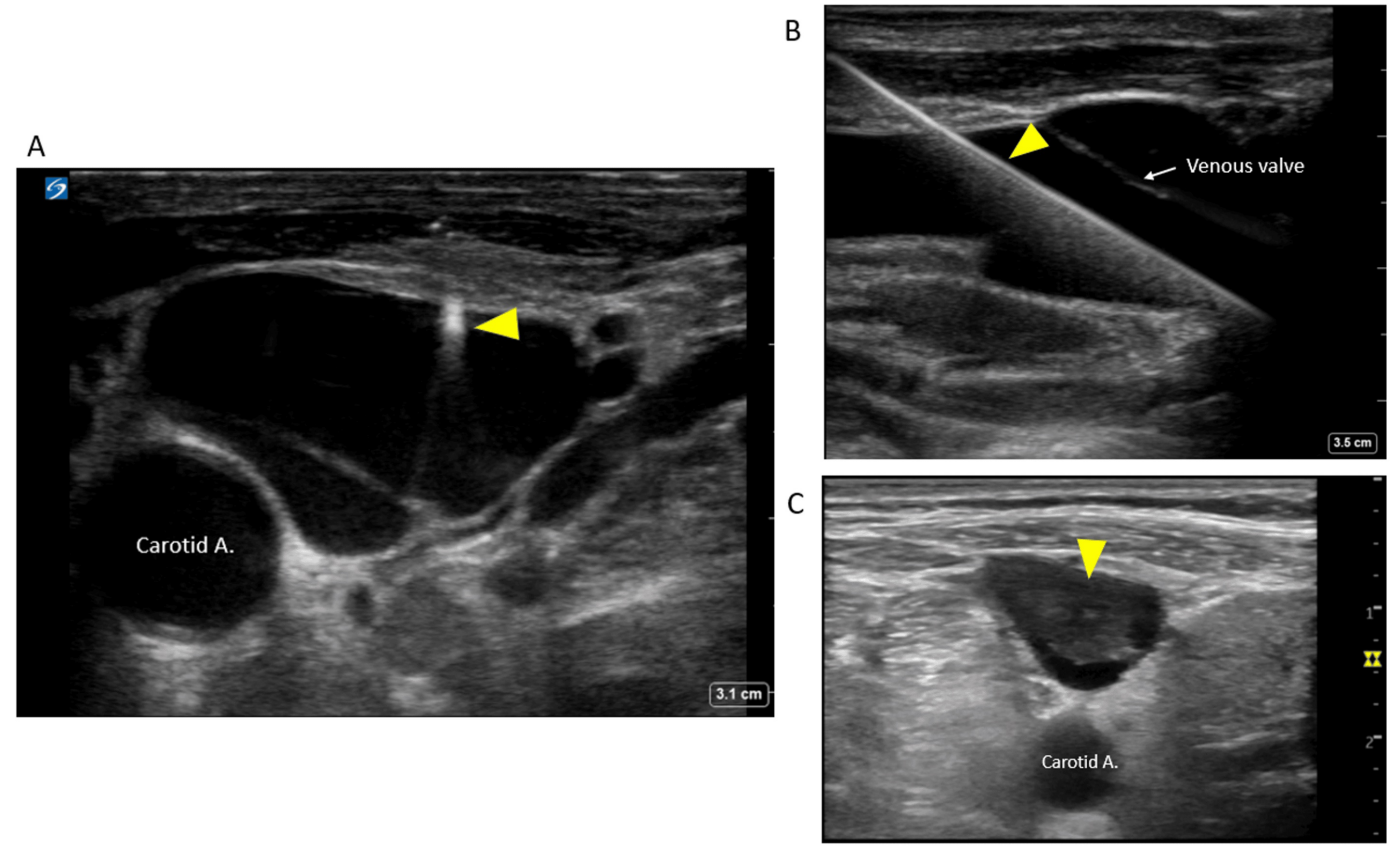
Ultrasound of the internal jugular vein for guiding central venous access placement (A) Needle tip in the vein (arrow). (B) Guidewire in the vein (arrow). (C) Thrombus (arrow) in the internal jugular vein detected prior to catheter placement. Image credit: Abhilash Koratala
